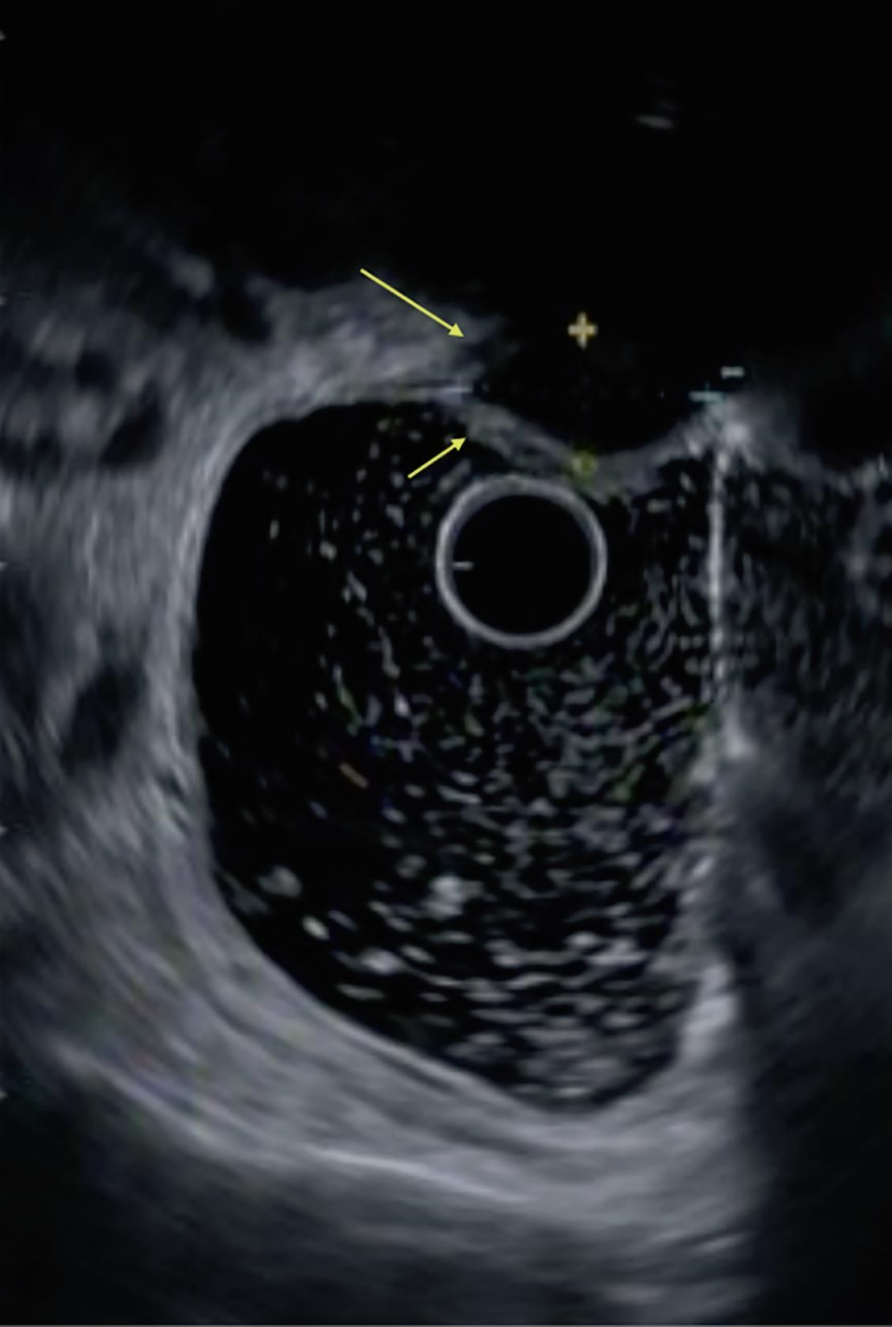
Rectal ultrasound demonstrating a 1.9 cm by 1.0 cm endometrial implant tethering the muscularis propria in the mid‐rectum without rectal invasion.
